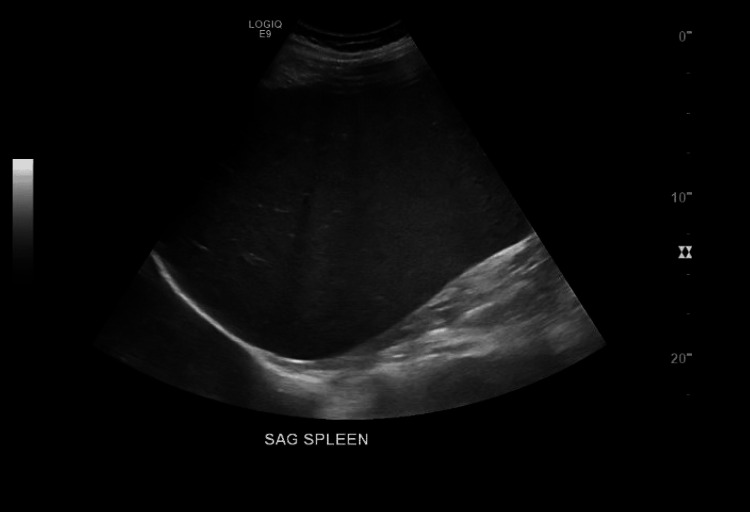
Abdominal ultrasound showing splenomegaly of 25 cm. SAG: sagittal
